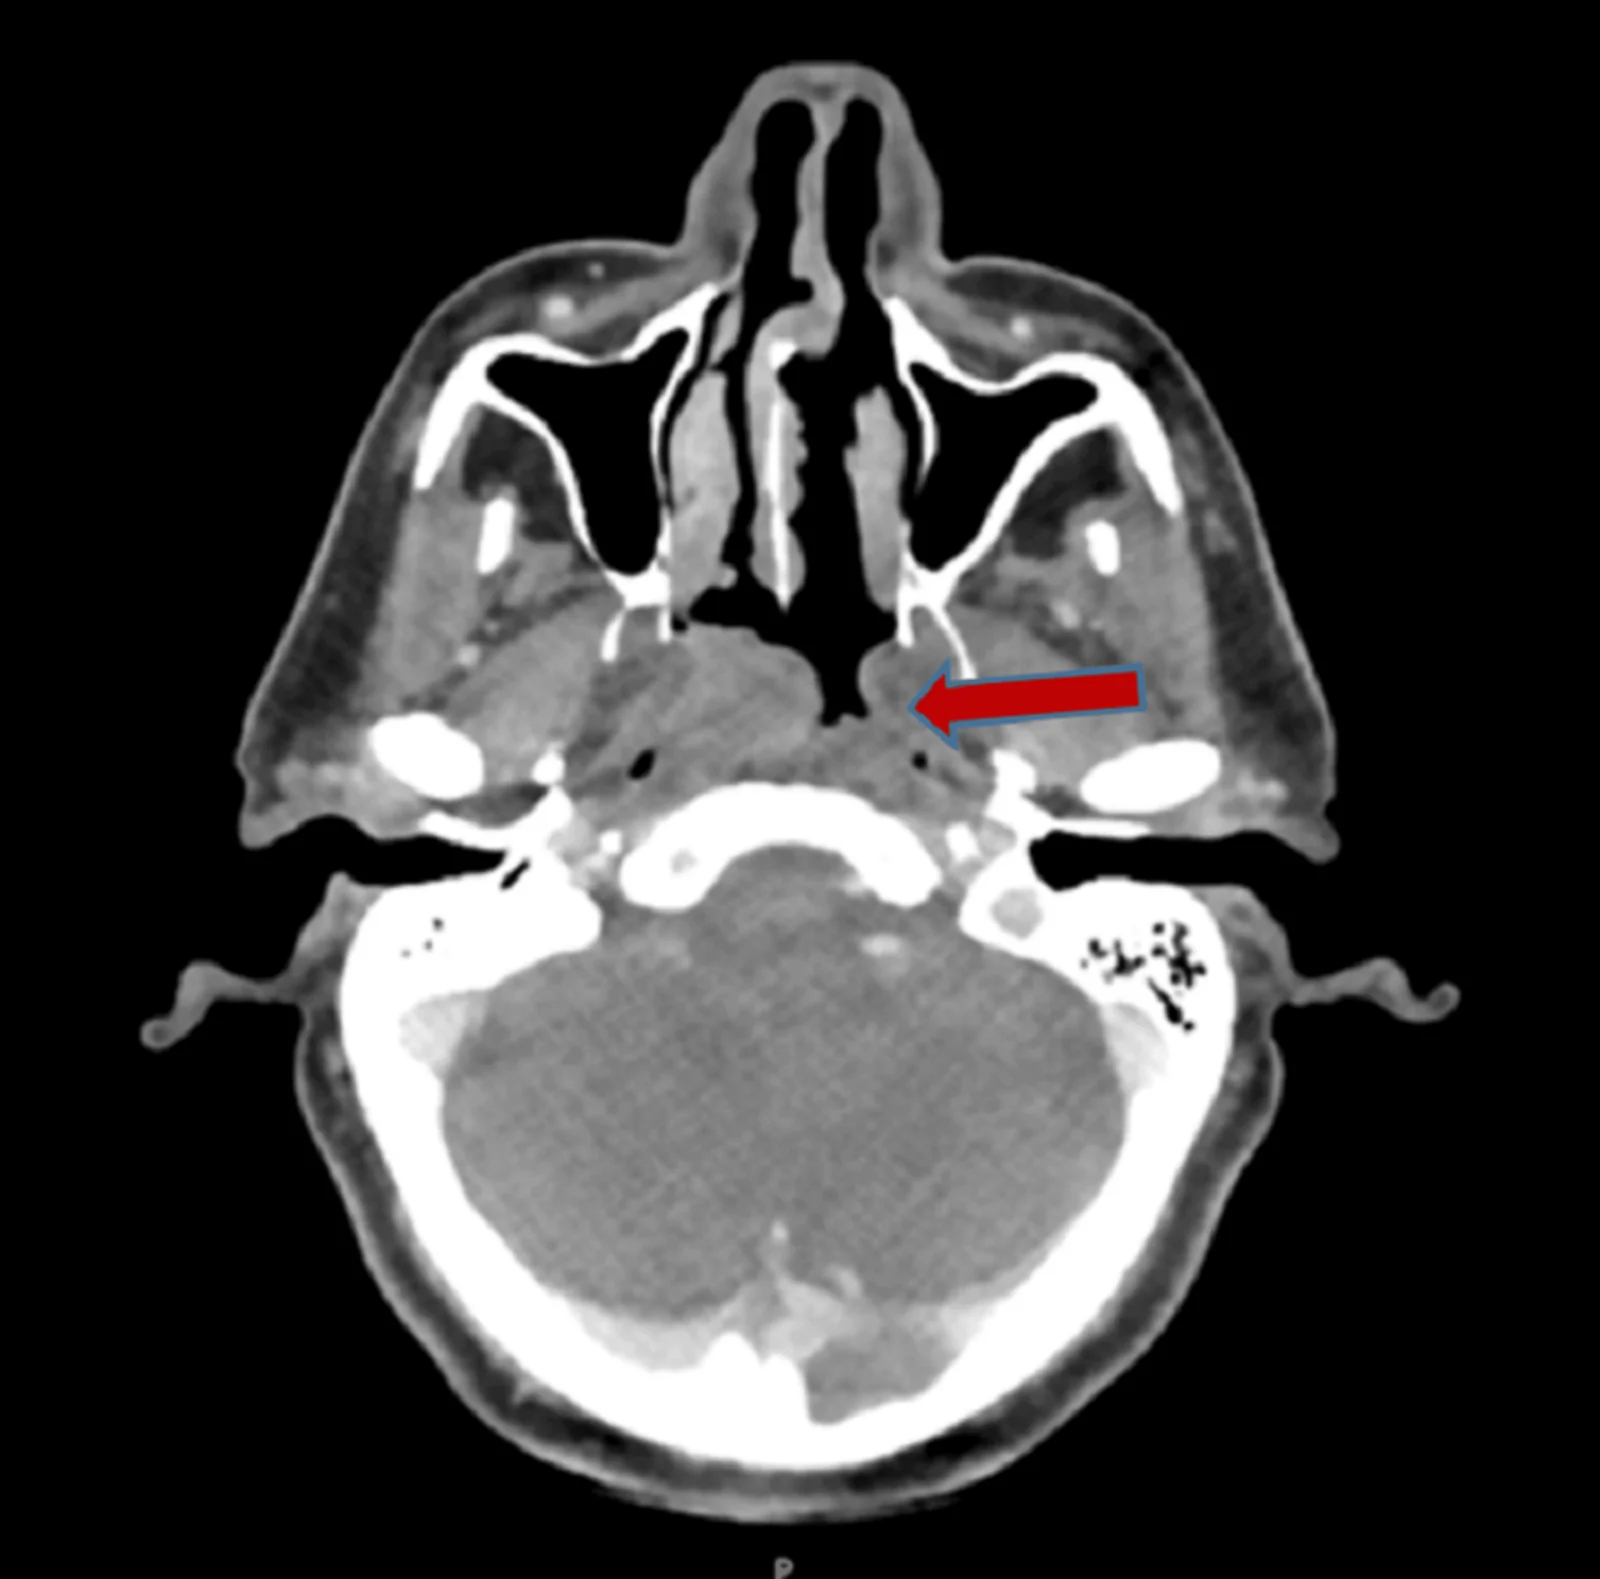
Computed tomography of the nasopharynx before treatment (arrow)
